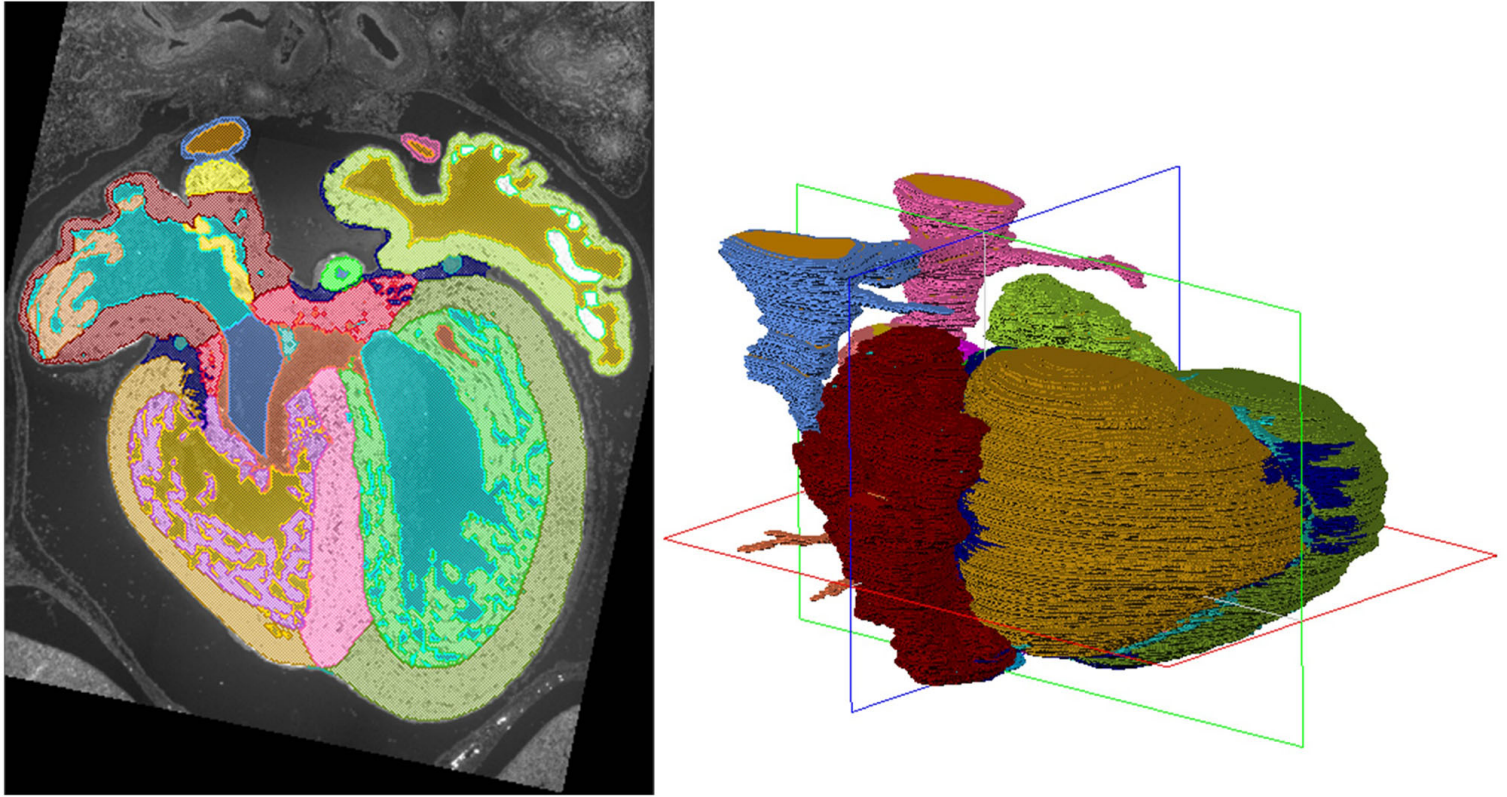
Making an ultrasound of a preserved human foetal heart with tricuspid atresia.
